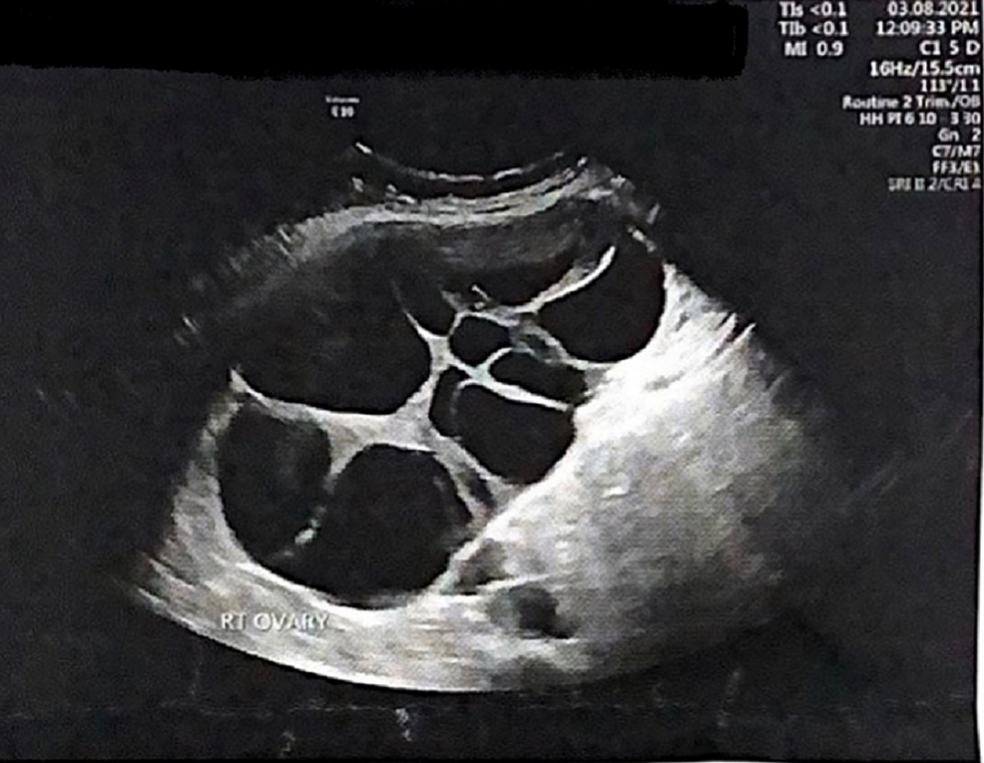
Right ovary Ultrasound scan of abnormally enlarged right ovary, depicting multiple cystic masses.
